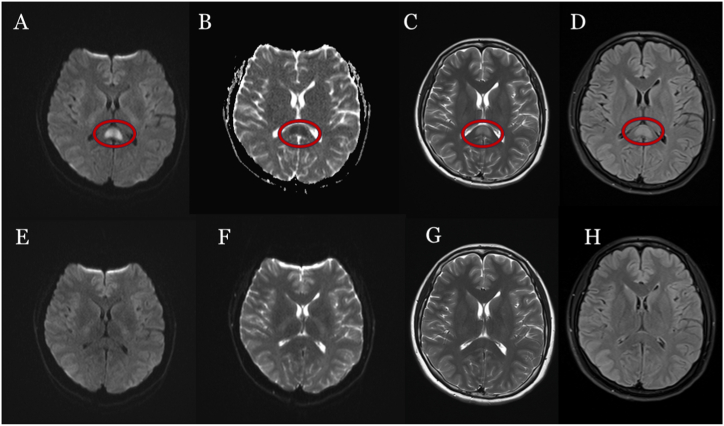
Brain MRI on admission and after treatment. (A–H) MR imaging reveals the SCC as hypersignal on diffusion-weighted imaging (DWI) (A) with a low apparent diffusion coefficient (ADC) value (B), and exhibiting high signal intensity on T2-weighted images (C) as well as on FLAIR images (D). Abnormalities detected on MRI completely resolved after 23 days (E–H).
